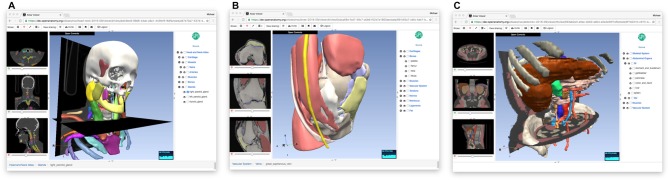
Other anatomy atlases. In addition to the SPL/neuroimage analysis center (NAC) Brain Atlas, we have converted three other atlases for use with OABrowser, featuring CT-derived atlases of the head and neck (A), the knee (B) and the abdomen (C).
